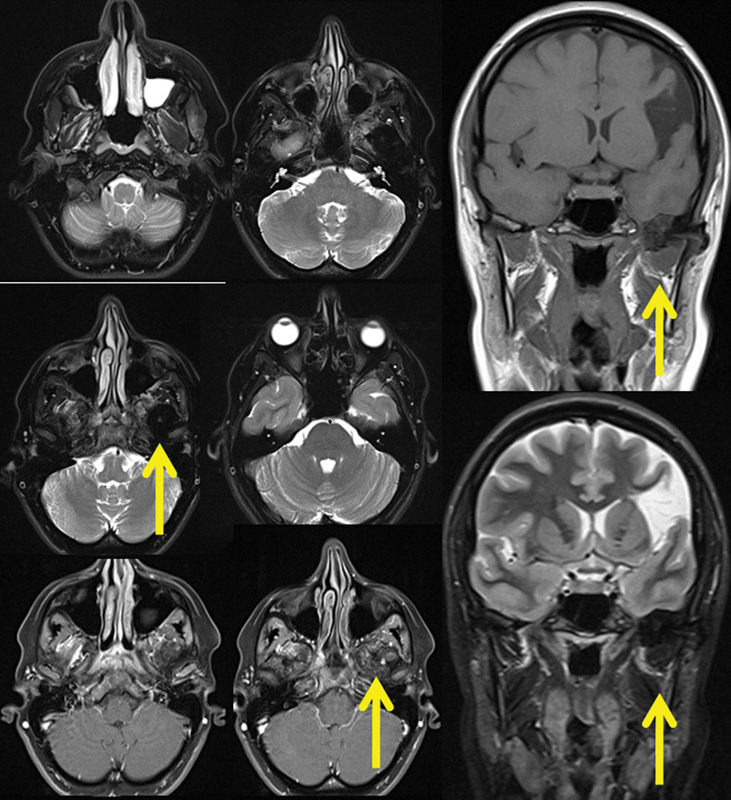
Magnetic resonance imaging of the skull identified a mass with low signal in T1- and T2-weighted images, with small focal enhancement with gadolinium.
